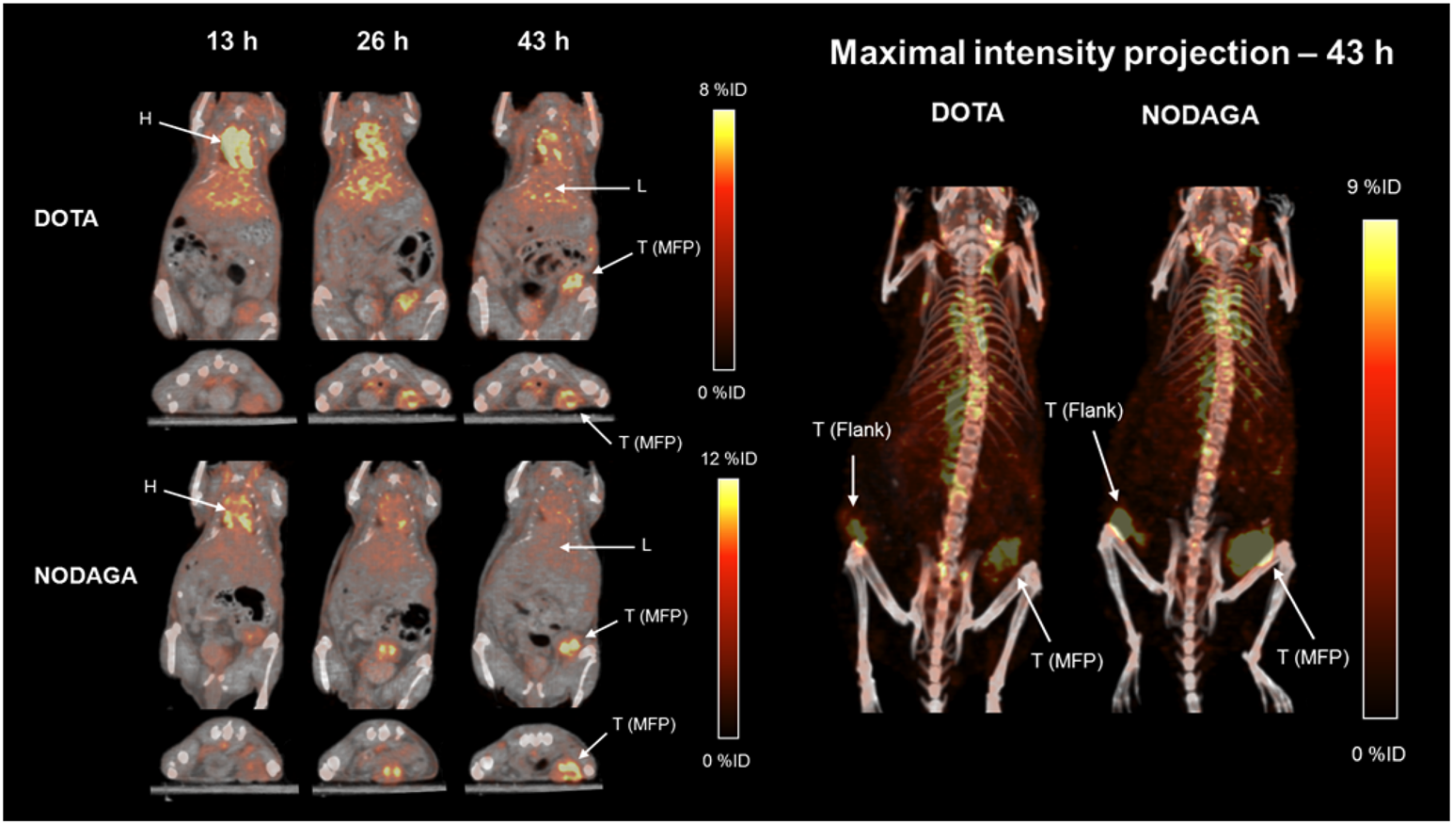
Coronal and axial PET/CT images of [^64^Cu]DOTA‐trastuzumab (DOTA) and [^64^Cu]NODAGA‐trastuzumab (NODAGA) 13, 26 and 43‐h post injection (left), and maximal projection images 43‐h post injection (right). H, heart; L, liver; T, tumour; MFP, mammary fad pad.
